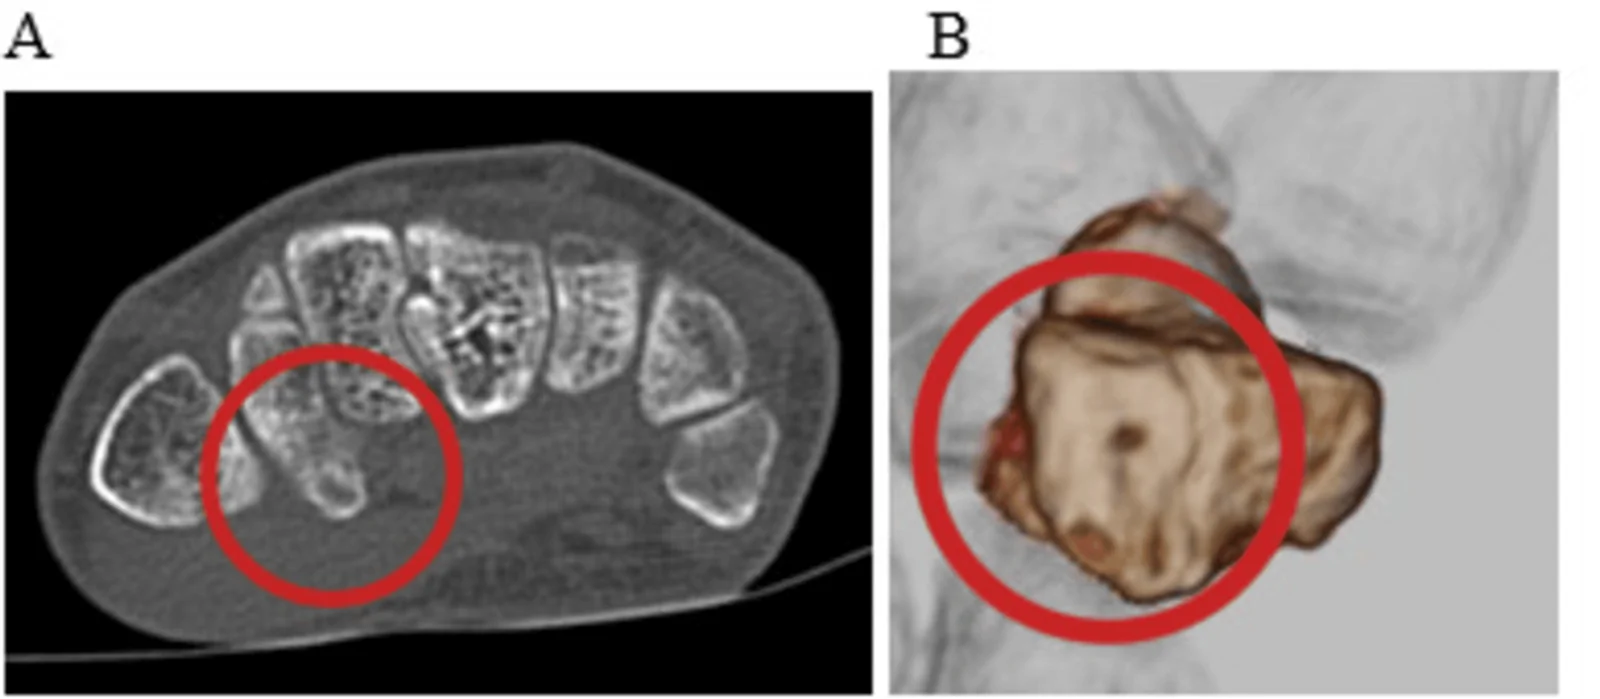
Case 1: CT images obtained six weeks post-injury. Axial (A) and three-dimensional (B) CT images showing callus formation with no exacerbation of the fracture (red circles).
